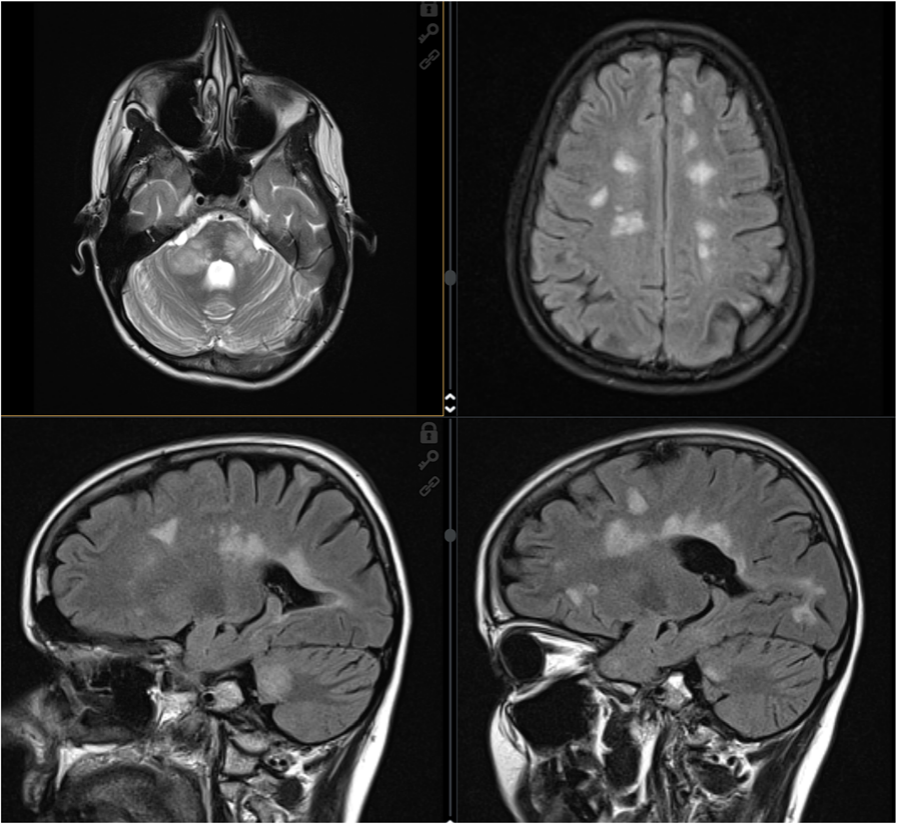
4/2019 – T2 and FLAIR images. Multiple MS lesions of supratentorial and infratentorial white matter
